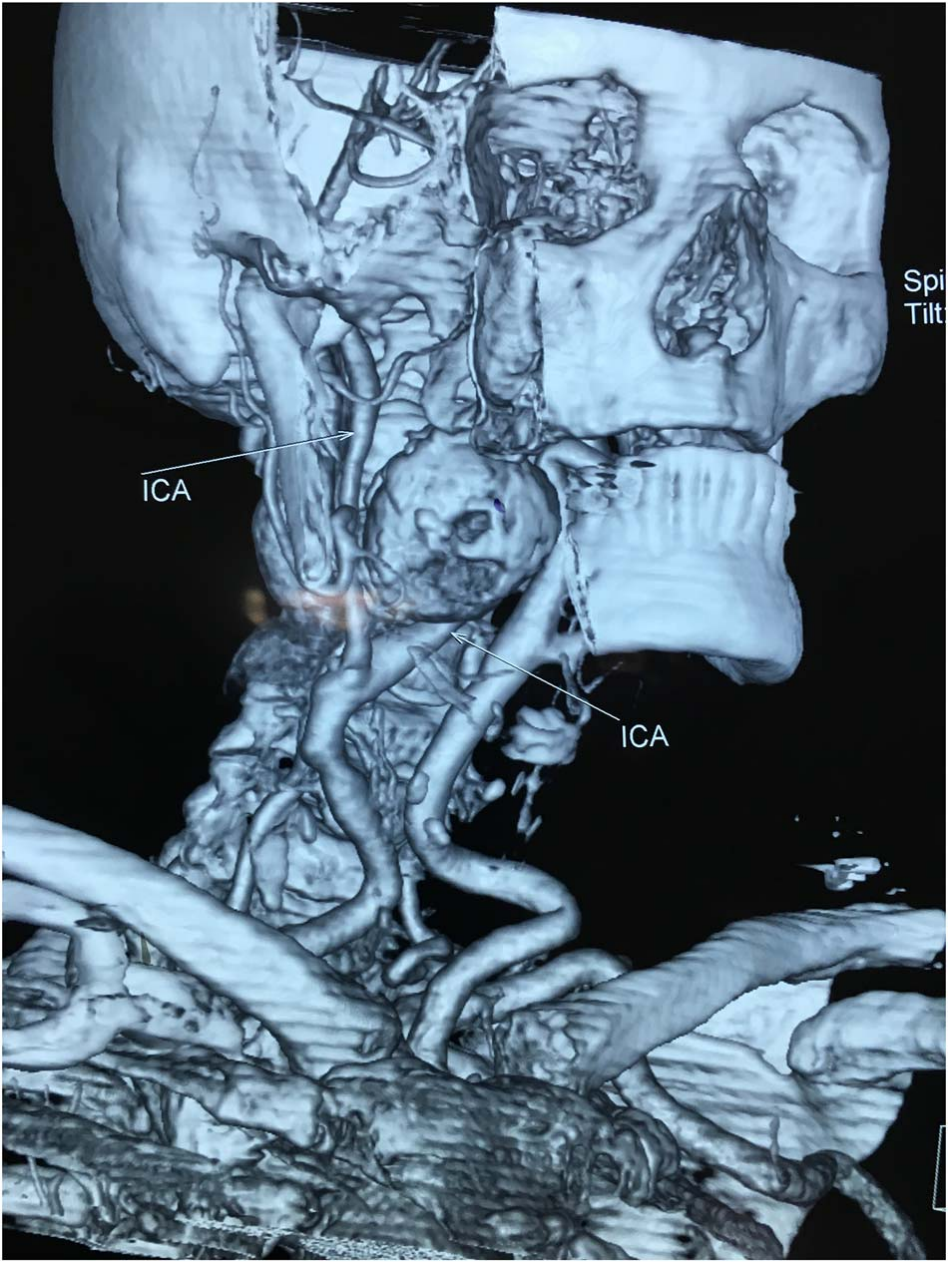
Computed tomography angiography of the carotids with 3D reconstruction.
